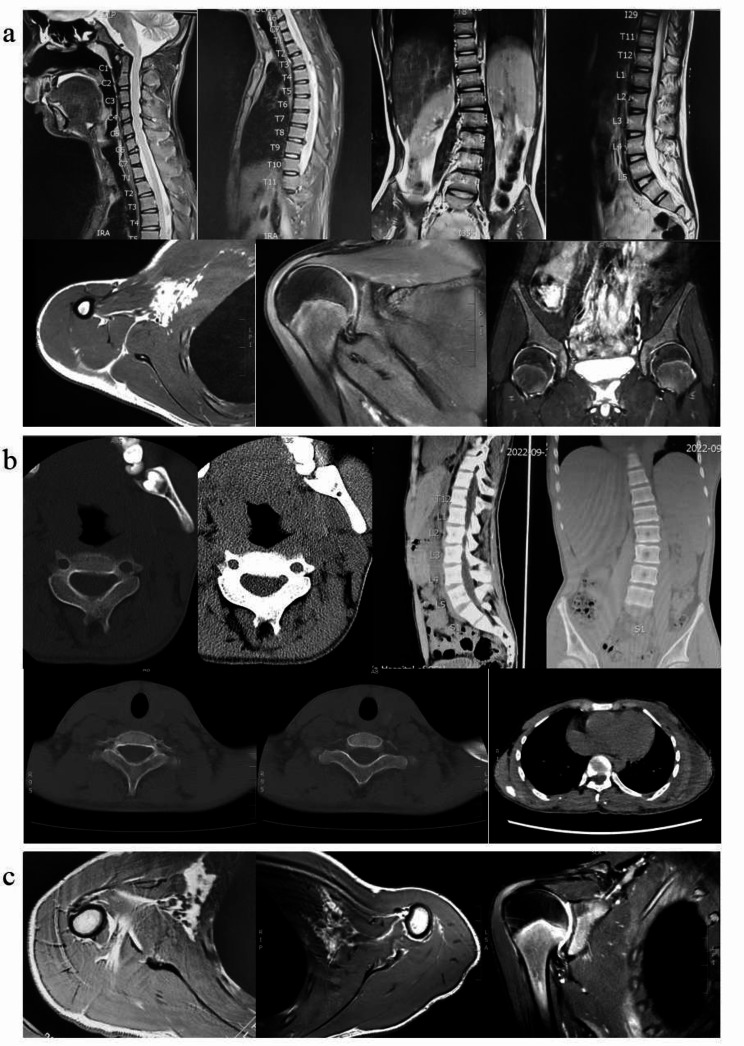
Imaging studies. (a) MRI of the first admission; (b) CT of the second admission; (c) MRI of the second admission
